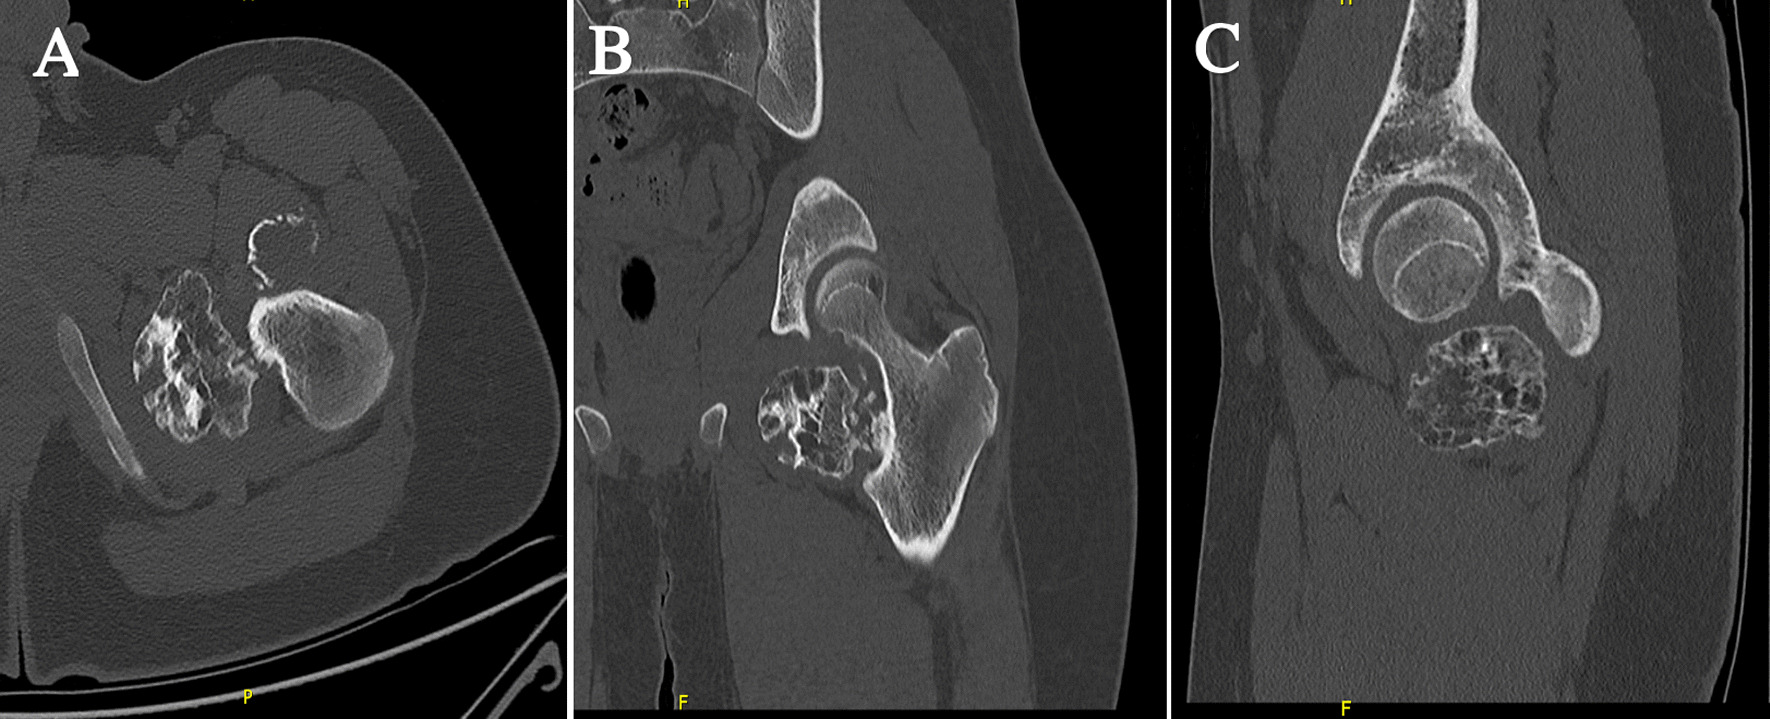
A Axial, B coronal, and C sagittal CT scans of the left hip showing the inferomedial lesion
